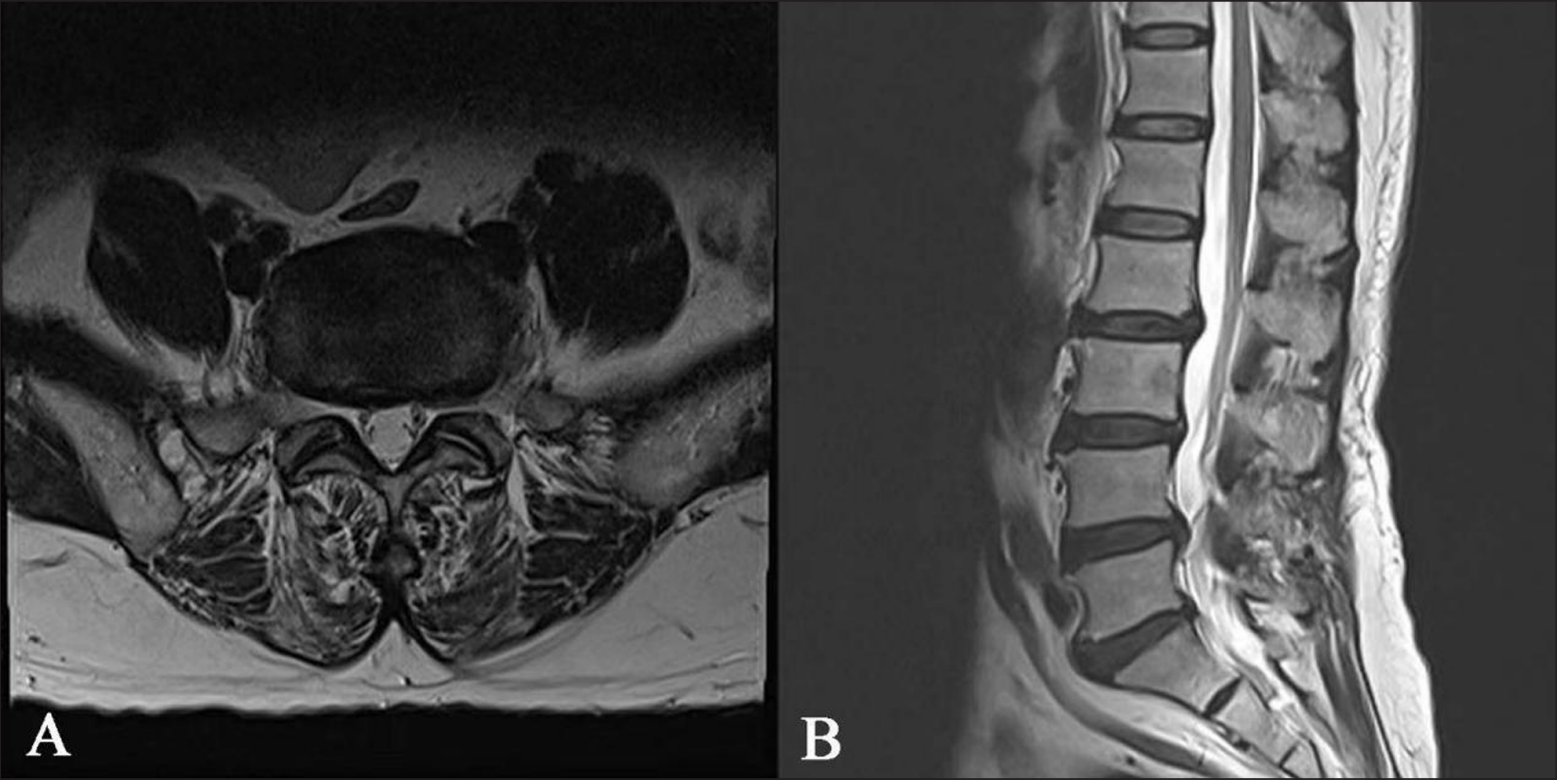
Preoperative: T2-weighted axial (A) and sagittal (B) MR images without contrast.
